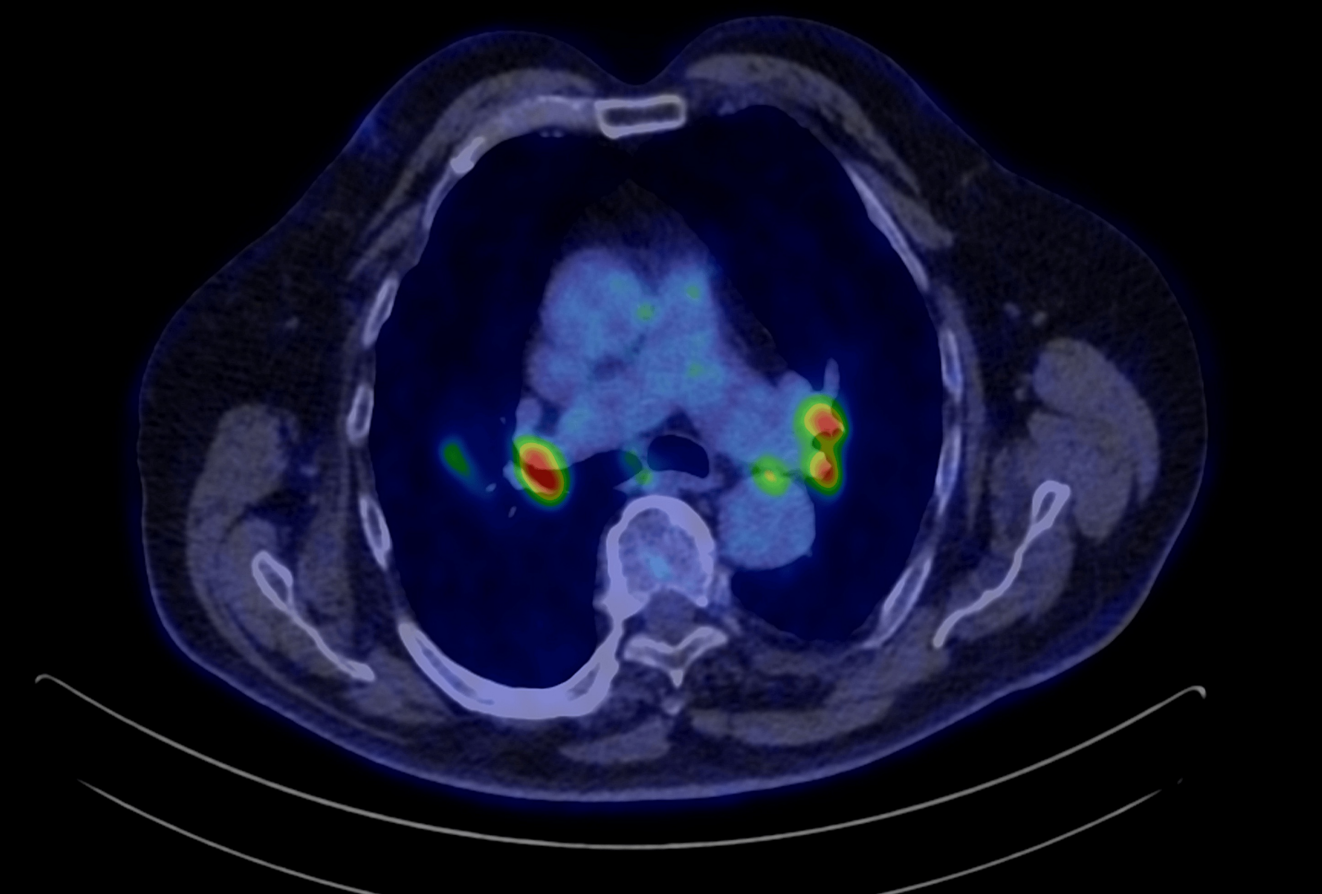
Axial 18F-fluorodeoxyglucose positron emission tomography/computed tomography (FDG-PET/CT) image. Axial-fused PET/CT image demonstrating bilateral mediastinal hypermetabolic lymph nodes with intense FDG uptake (SUVmax up to 12.4).
